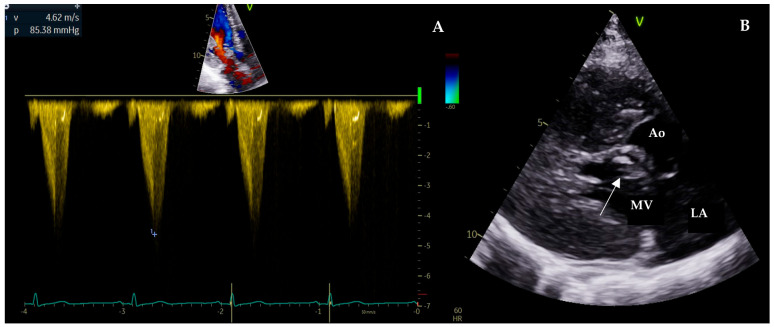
(A) LVOT dynamic gradient (85 mmHg, Vmax 4.62 m/s) measurement with the continuous doppler by TTE (number 1). (B) Presence of SAM of the mitral valve (arrow) assessed by TTE. Ao: Aorta; LA: Left atrium; LVOT: Left ventricle outflow tract; MV: Mitral valve; SAM: Systolic anterior movement; TTE: Transthoracic echocardiography; V: Velocity.
